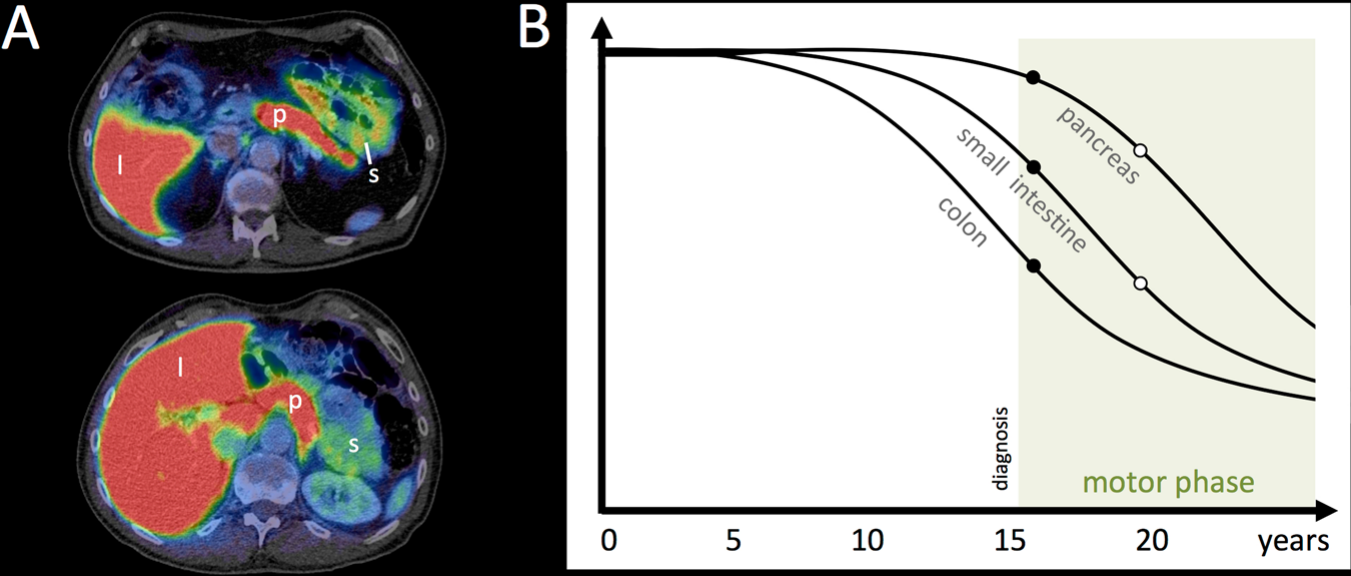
^11^C-donepezil PET/CT images. a Summed PET images superimposed on anatomical CT in a healthy control (*top*) and a PD patient (*bottom*). Note the visually apparent decrease in the small intestine signal. b Hypothetical timeline of parasympathetic denervation during the course of prodromal and manifest PD. *Closed* and *open circles* represent ^11^C-donepezil PET data from de novo (duration 1 year) and moderate stage PD patients (duration 4.5 years), respectively.^65, 66^ Shortly after diagnosis, some ^11^C-donepezil signal loss is seen in the small intestine and colon, but not in the pancreas. Five years after diagnosis, progressive signal loss is seen in the pancreas, small intestine, and colon. [l liver, p pancreas, s small intestine]
